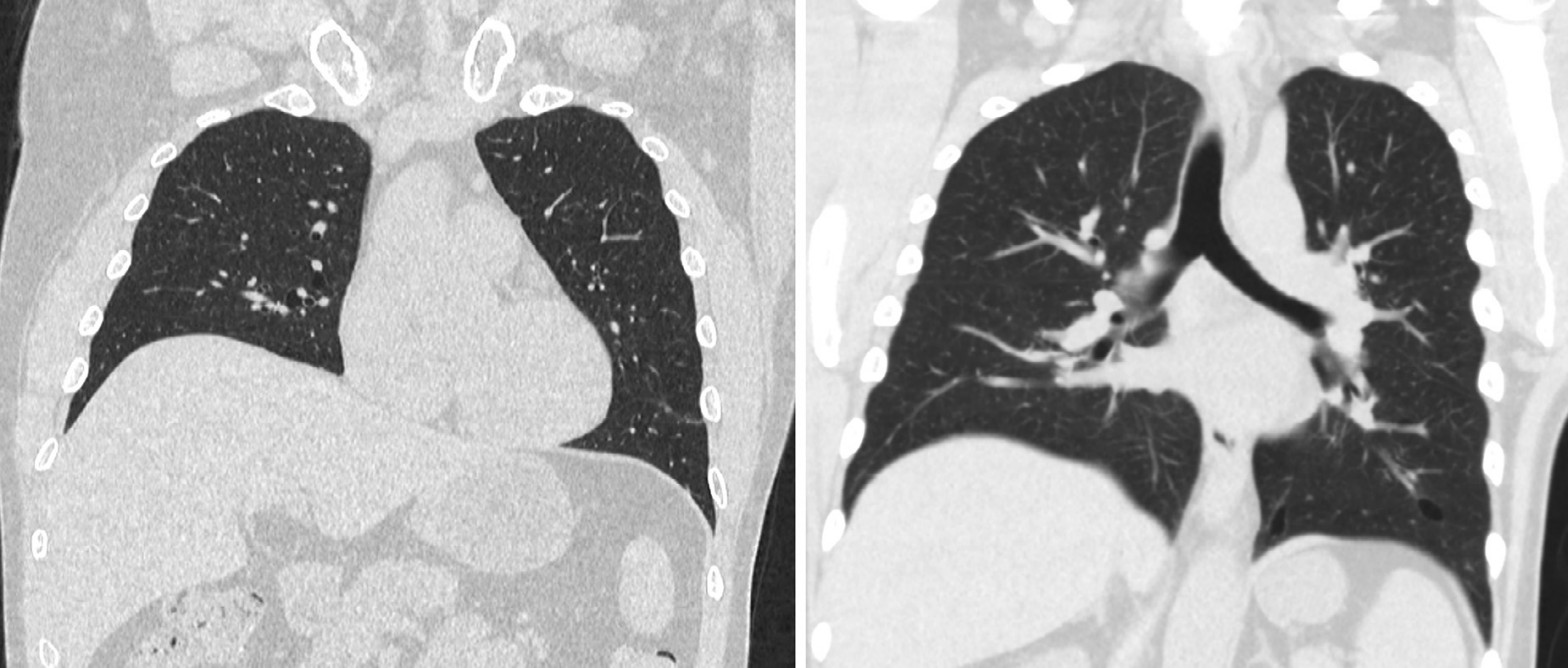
CT Chest without contrast: Coronal images demonstrate multiple thin-walled elliptical well-defined para-mediastinal air-filled cysts in a basilar distribution with preserved lung volume and no evidence of interstitial lung disease
